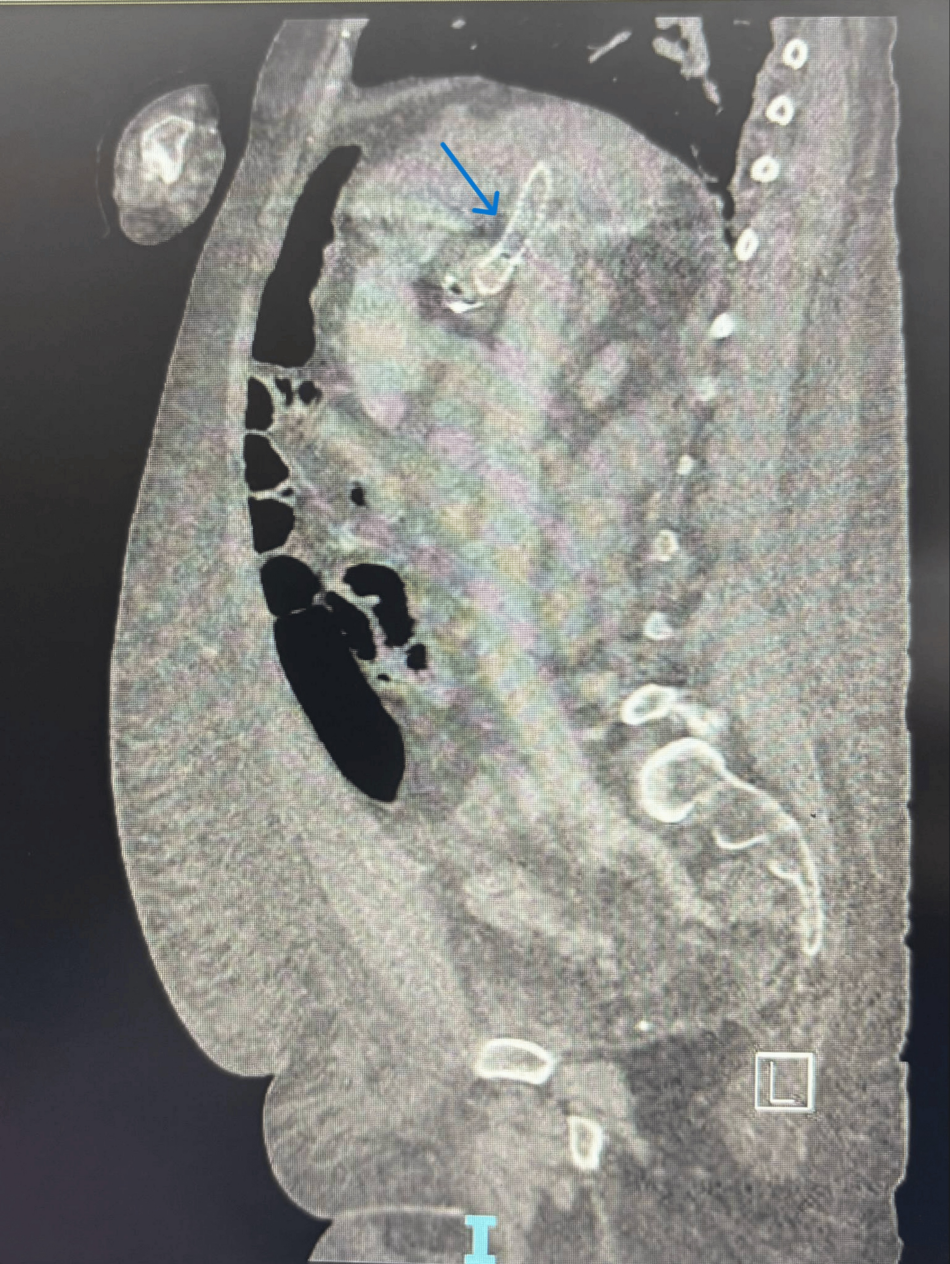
CT chest, abdomen, and pelvis - sagittal view featuring patent TIPS CT: computed tomography; TIPS: transjugular intrahepatic portosystemic shunt
